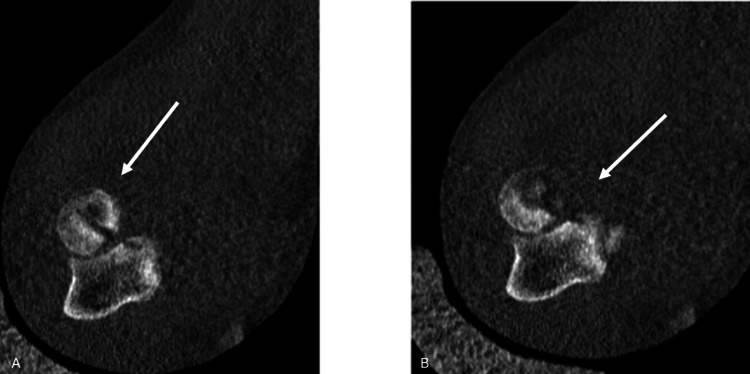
Axial section of the computed tomography images of the patient. Both A and B demonstrate the fracture in the radial head White arrows indicate the fracture at proximal radius.
